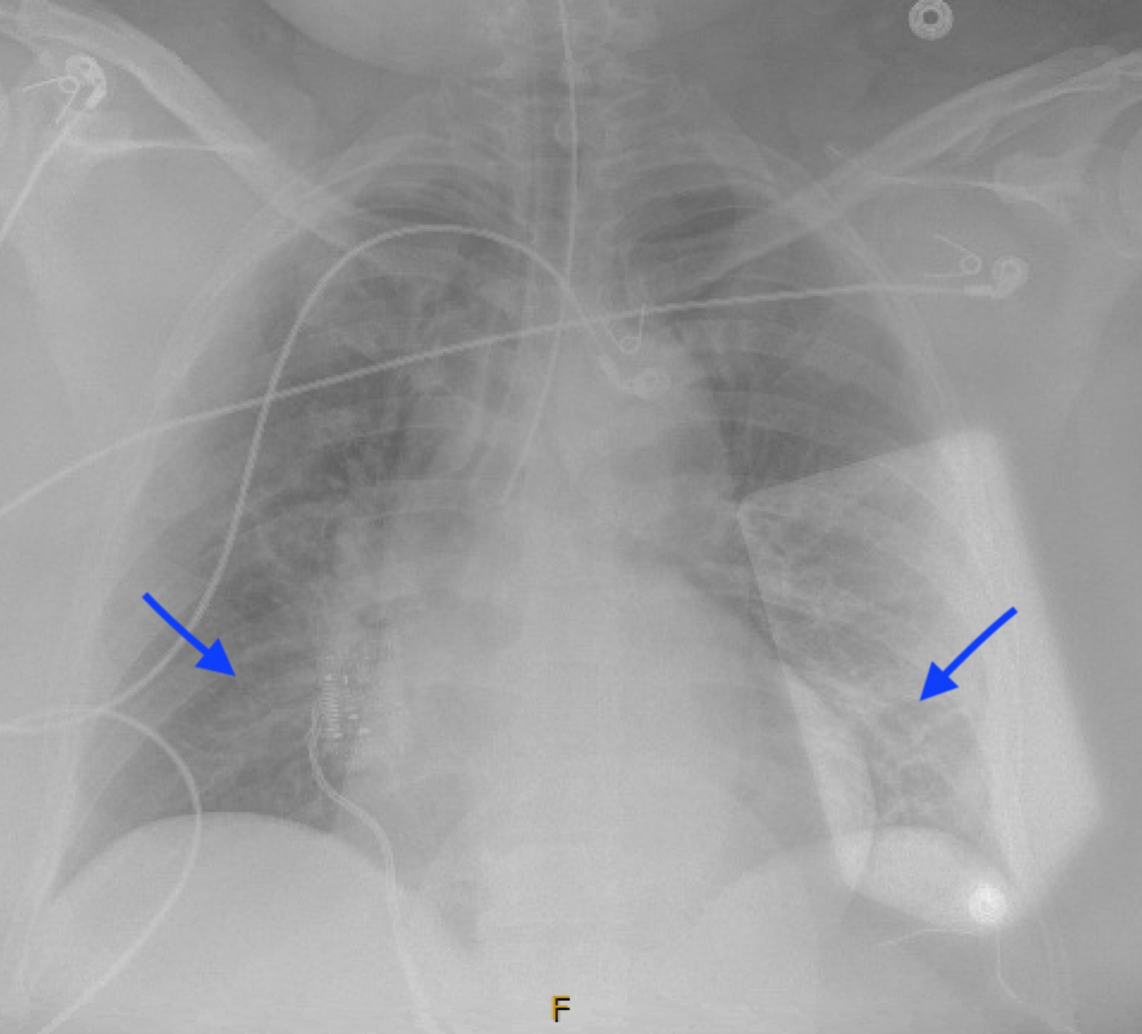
Portable chest X-ray Chest X-ray seven hours after admission, showing worsening bilateral patchy infiltrates.
